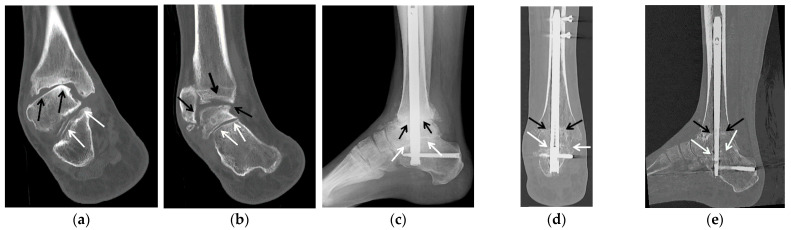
(a–e) A 58-year-old male with tibiotalar and subtalar osteoarthritis with varus alignment treated with tibiotalocalcaneal arthrodesis with intramedullary nail and iliac crest bone marrow aspirate. (a,b) Coronal reformatted preoperative CT images of the right ankle show advanced osteoarthritis of the tibiotalar (black arrows) and posterior subtalar (white arrows) joints with associated varus deformity. (c) Six weeks postoperative lateral weight-bearing radiograph shows a retrograde tibiotalocalcaneal intramedullary nail with interlocking screws in the calcaneus and distal tibial diaphysis transfixing the subtalar (white arrows) and tibiotalar (black arrows) joints with associated bone graft material. (d) Coronal and (e) sagittal reformatted CT images obtained 2.5 months after surgery show markedly improved alignment with progressive fusion across the subtalar (white arrows) and tibiotalar (black arrows) joints.
